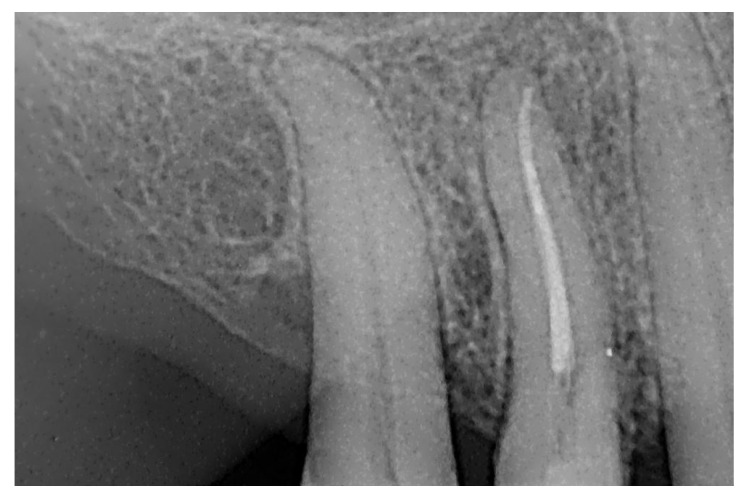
Intraoral radiography of tooth 1.4 at 12-month follow-up.
